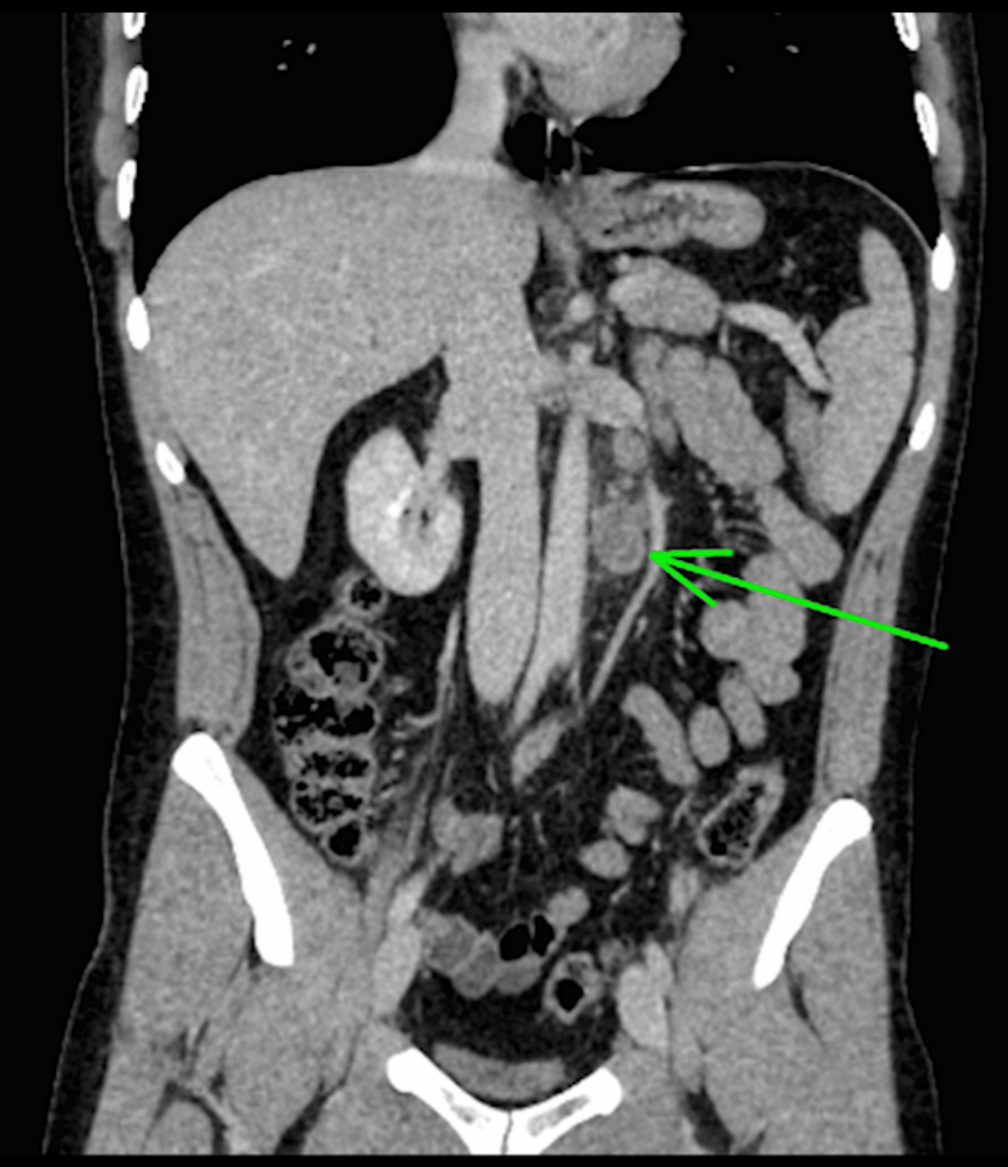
Coronal computed tomography scan of the abdomen showing para-aortic lymphadenopathy
